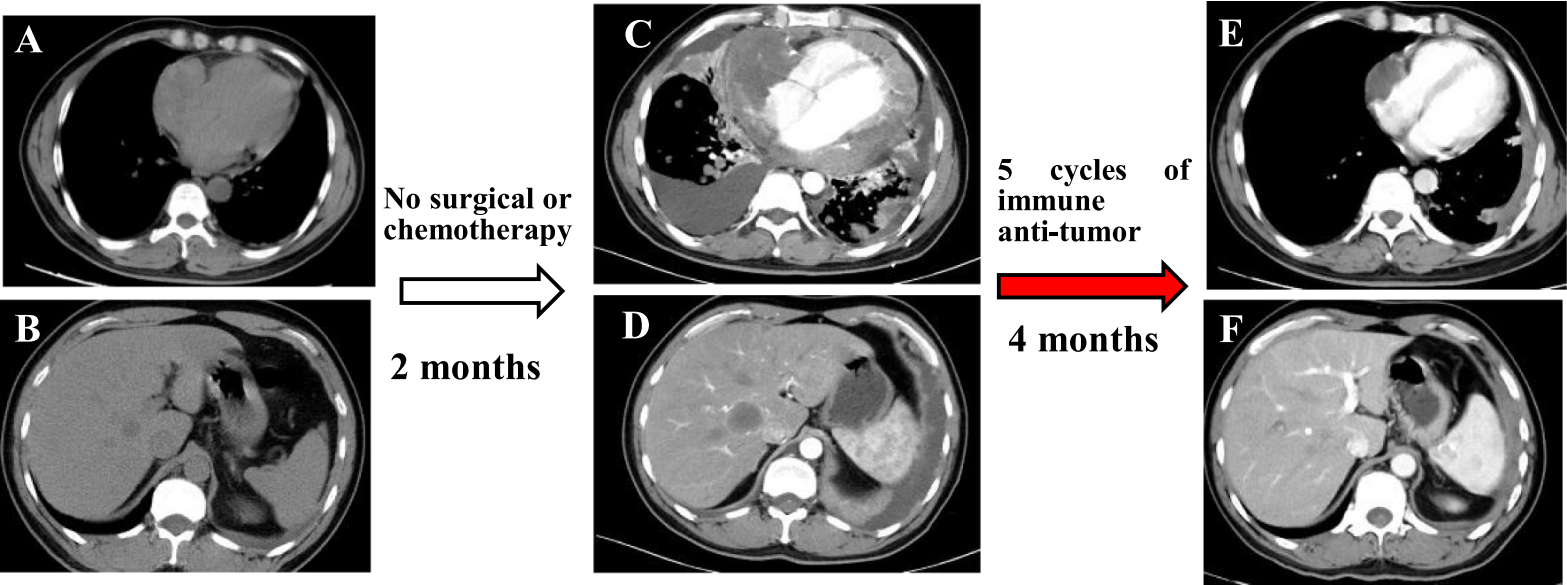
CT imaging displayed right atrium mass (a) and suspected metastases in liver (b). Scan enhancement CT scan show abnormal signals in the right atrium, pericardium (c), and liver (d) in 2 months. After 5 cycles of comprehensive immune anti-tumor therapy, significant reduction is detected in the right atrial intimal sarcoma (e) and liver mass (f) dimension
